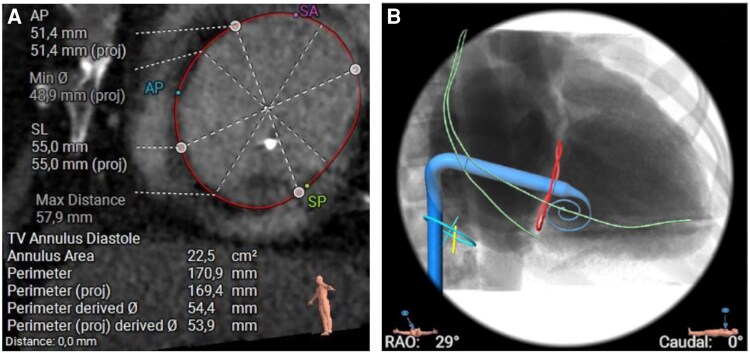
Transthoracic view of the triscupid valve after Evoque implantation showing the prosthesis in loco typico with minimal paravalvular leakage and a slightly increased mean pressure gradient (4.68 mmHg).
